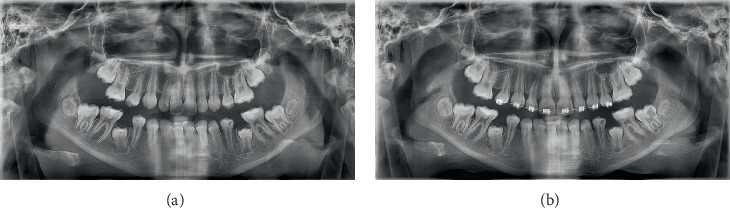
(a) Panoramic radiograph (January 2023): distal radiolucency in Tooth 37 without pulp involvement. (b) Panoramic radiograph (May 2023): rapid lesion progression with potential pulp involvement.
